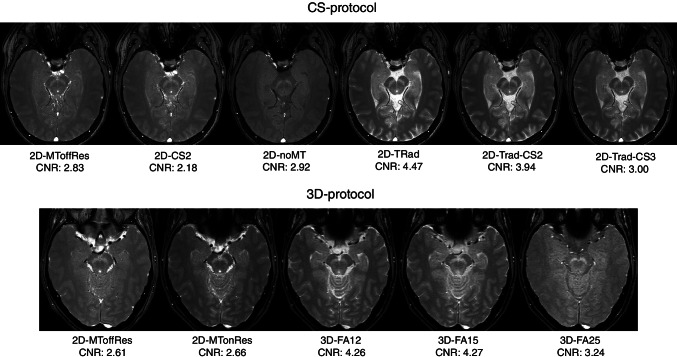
Overview of the neuromelanin MRI scans. An example of the neuromelanin MRI sequences of one participant of the CS protocol and one participant of the 3D protocol, with the mean contrast to noise ratio (CNR) of all participants per scan. MT, magnetization transfer; Res, resonance; CS, compressed sense; TRad, repetition time adjusted; FA, flip angle
